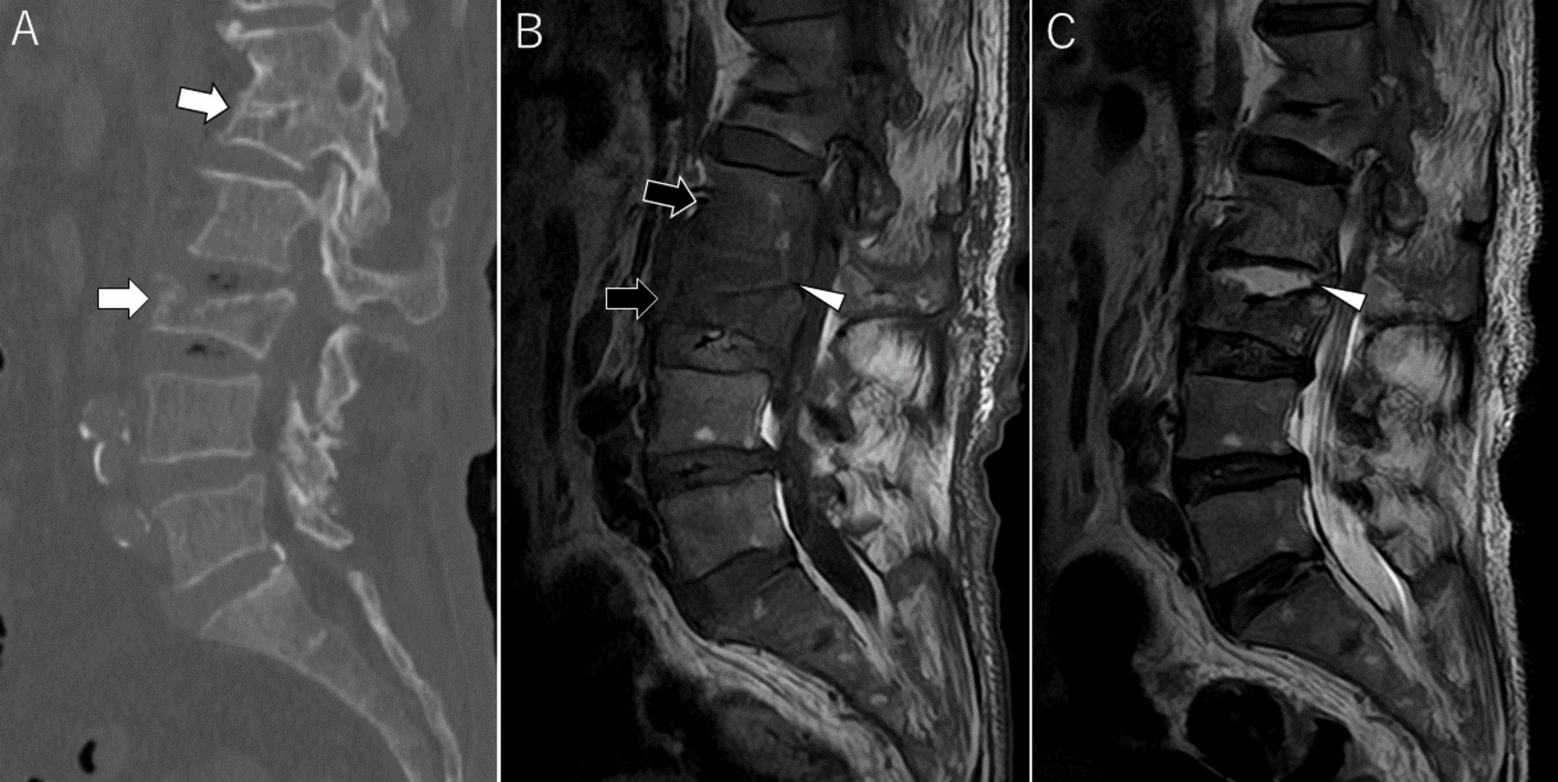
Lumbar Spine Computed Tomography (CT) and Magnetic Resonance Imaging (MRI) Lumbar CT (A) reveals compression fractures of the first and third lumbar vertebral bodies (white arrows). T1-weighted imaging (T1WI) (B) shows hypointensity in the second and third lumbar vertebral bodies (black arrows) and irregular margins with low signal intensity in the L2/3 intervertebral disc. T2-weighted imaging (T2WI) (C) shows hyperintensity in the L2/3 disc (arrowhead). These findings are suggestive of pyogenic spondylitis.
